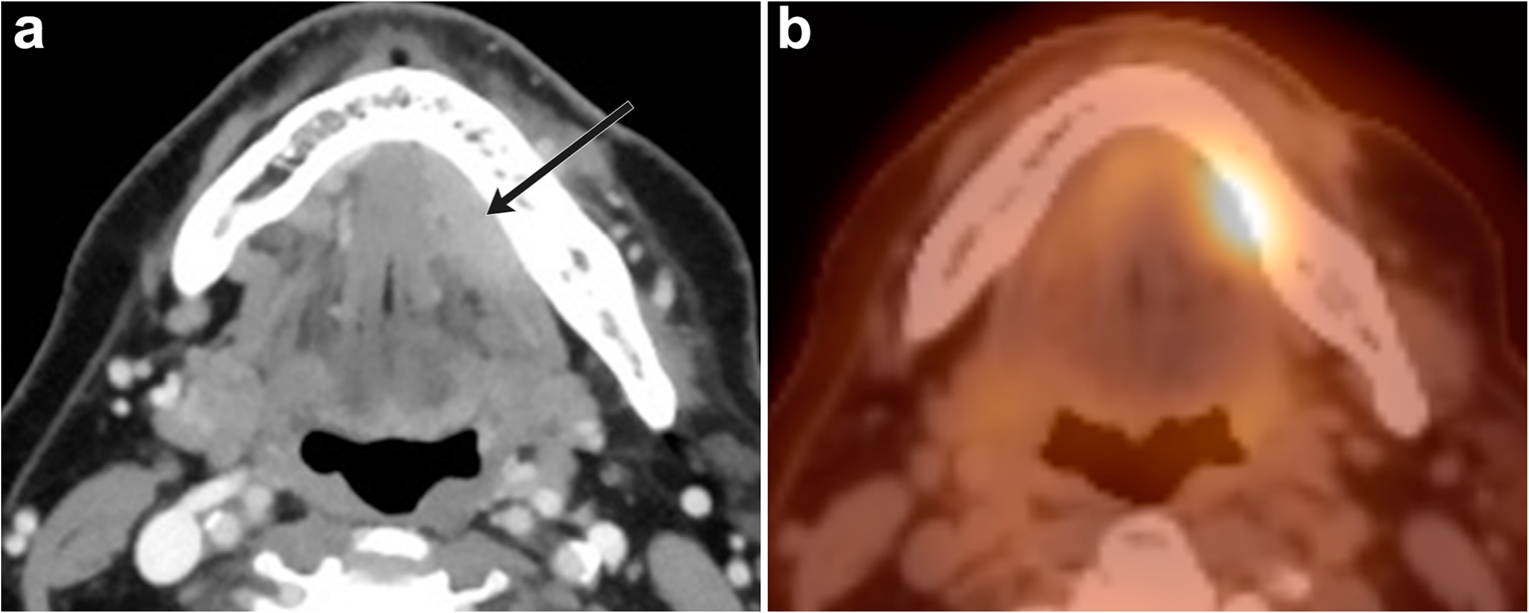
Squamous cell carcinoma involving the left sublingual space. a Axial contrast-enhanced CT image shows a homogenously enhancing lesion within the left sublingual space (*arrow*), b which demonstrates ^18^F-fluorodeoxyglucose (FDG) avidity on PET/CT
